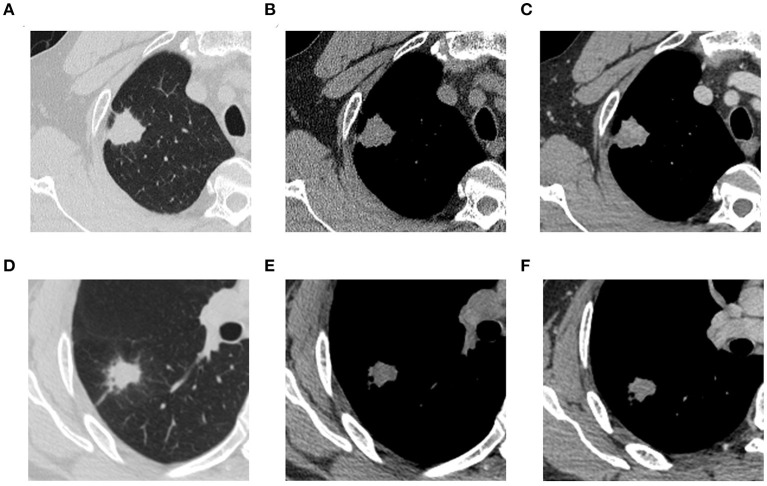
(A) (NCECT, lung window), (B) (NCECT, mediastinal window), and (C) (CECT, mediastinal window) are thoracic CT images of a 53-year-old male patient with pathologically confirmed TB. (D) (NCECT, lung window), (E) (NCECT, mediastinal window), and (F) (CECT, mediastinal window) are thoracic CT images of a 41-year-old male patient with pathologically confirmed organized pneumonia. TB, tuberculosis; NCECT, non-contrast enhanced CT; CECT, contrast-enhanced CT.
